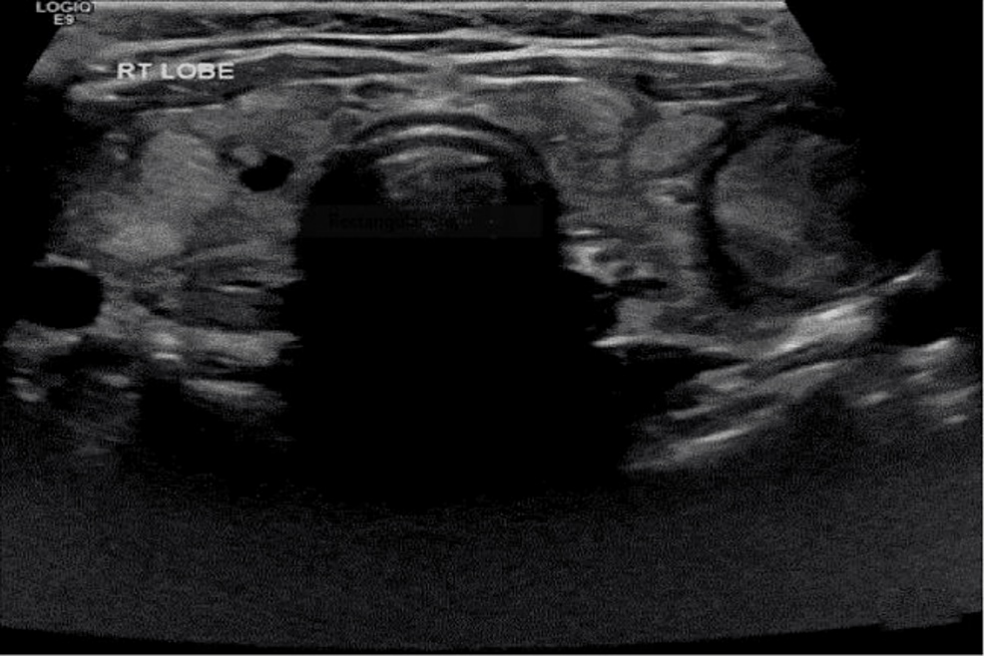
Thyroid ultrasound of Case 2 showing multinodular goiter with varying sizes of nodules.
